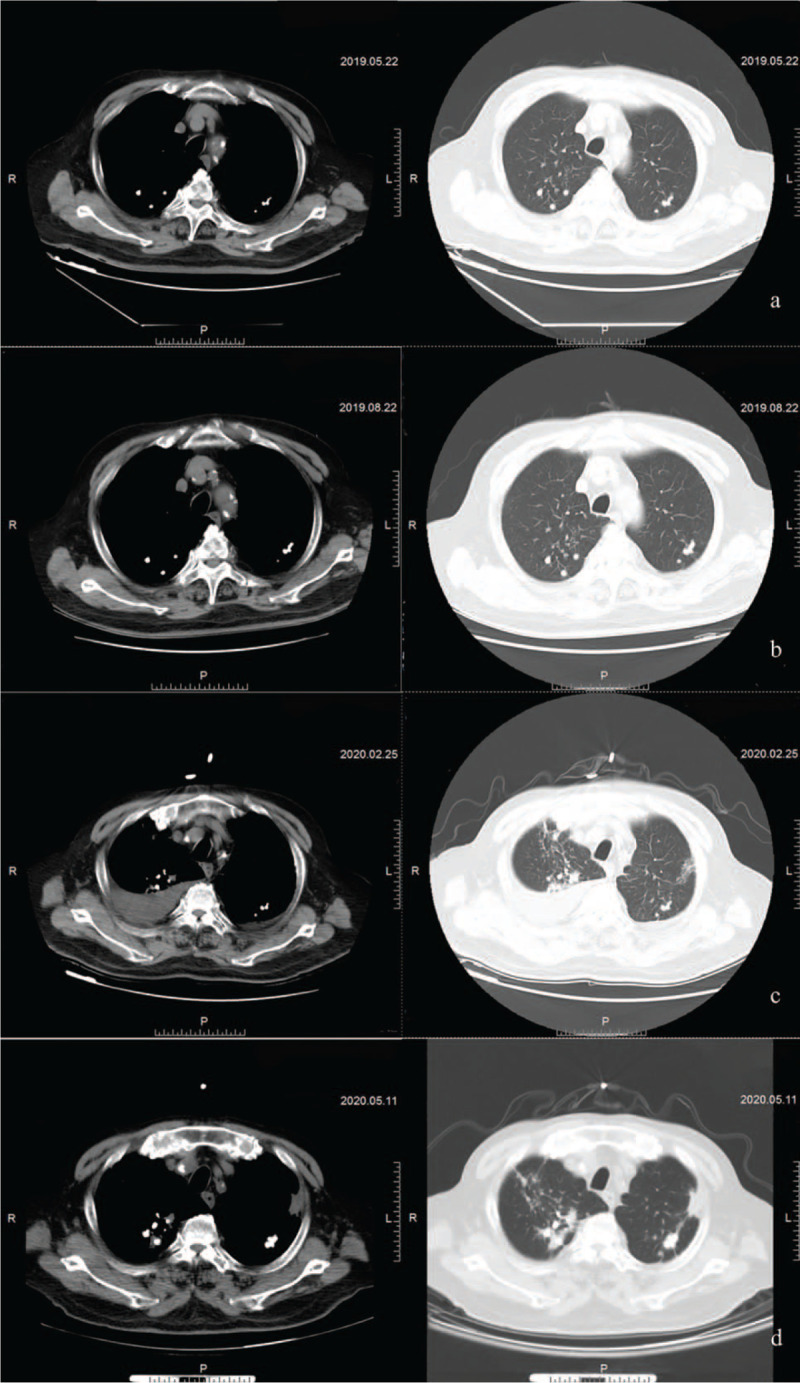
Chest computed tomography (CT) during treatment. (A) After 5 courses of docetaxel plus prednisone chemotherapy, the chest CT indicated that mild interstitial changes with a small amount of exudation, fibrous streak shadow, and signs of old pulmonary tuberculosis in both lungs. (B) After 3 courses of abiraterone plus prednisone, the chest CT displayed both lungs were the same as before. (C) Recurrence of tuberculosis after nine courses of abiraterone plus prednisone, the chest CT exhibited bilateral pleural effusion with atelectasis on February 25, 2020. (D) After 2 courses of anti- tuberculosis (TB) combine with abiraterone plus prednisone (5 mg/d), the pleural effusion were completely absorbed in both lungs.
